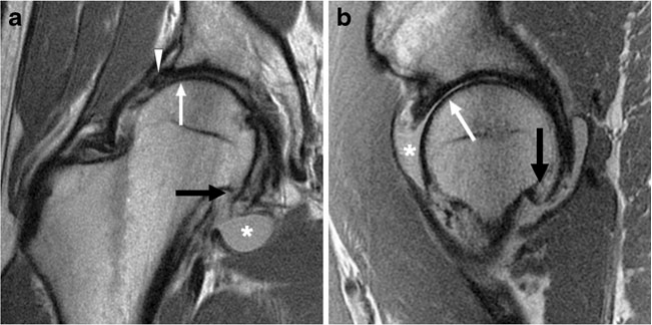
A 36-year-old man with cam-type FAI and severe osteoarthritis. Coronal (a) and sagittal (b) PD-weighted MR images of the right hip demonstrate chronic degeneration of the labrum (*arrowhead*). Chondral loss with extensive bone-on-bone contact is seen over the superior femoral head (*white arrow*). Subcapital femoral neck osteophytes are also seen (*black arrow*). Reactive synovitis with effusion is demonstrated (*asterisk*)
